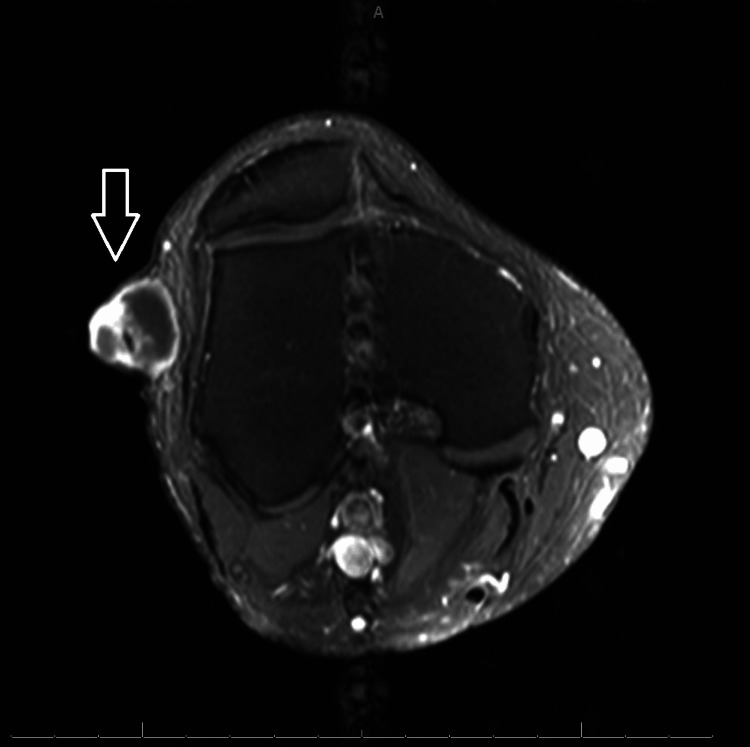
Axial MRI of the right knee (T1-weighted, post-contrast) demonstrating peripheral contrast enhancement along the laterally located lesion (arrow). MRI, magnetic resonance imaging
